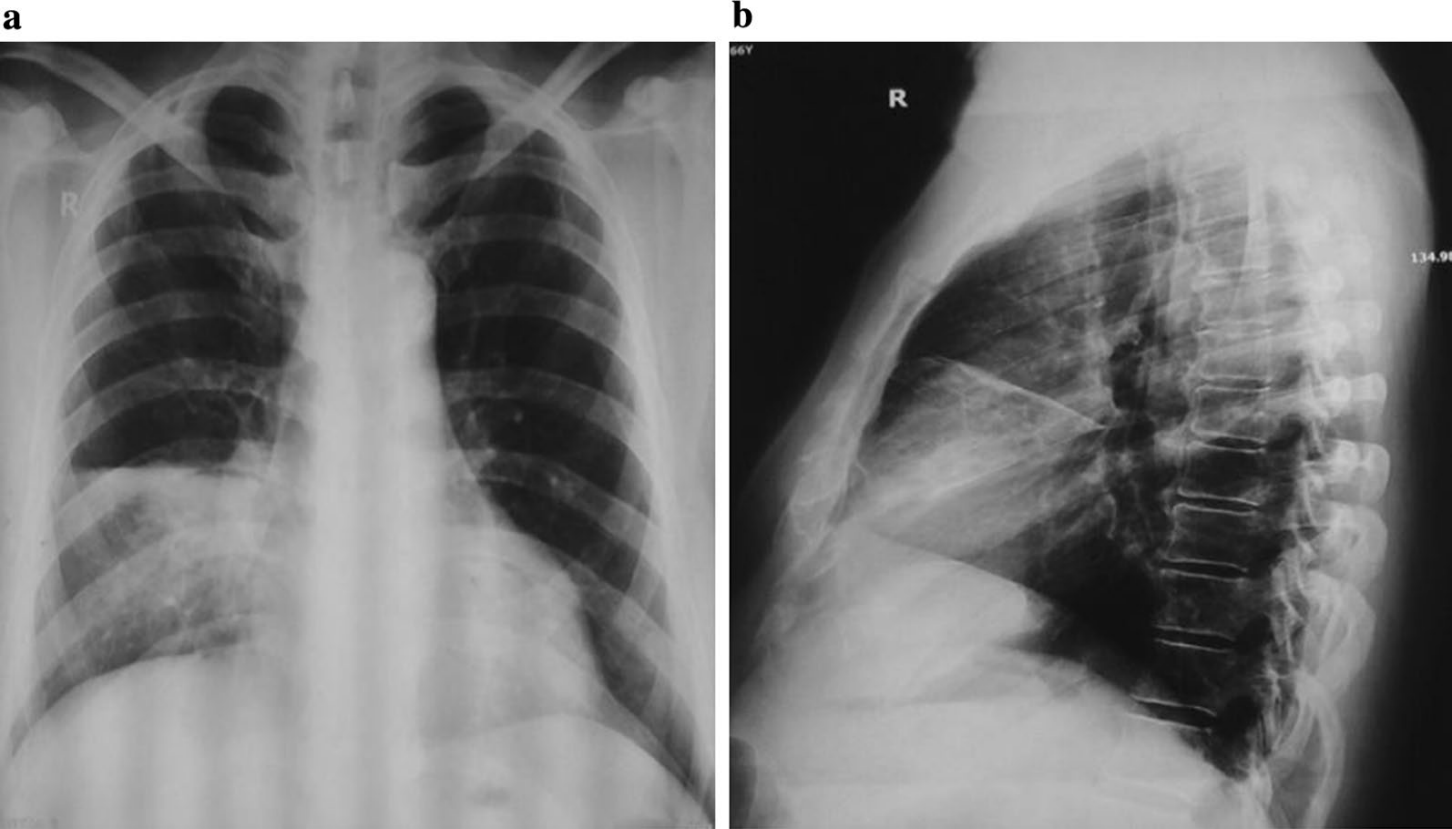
Chest X-ray (a) postero-anterior and (b) right lateral view showing right middle lobe consolidation
